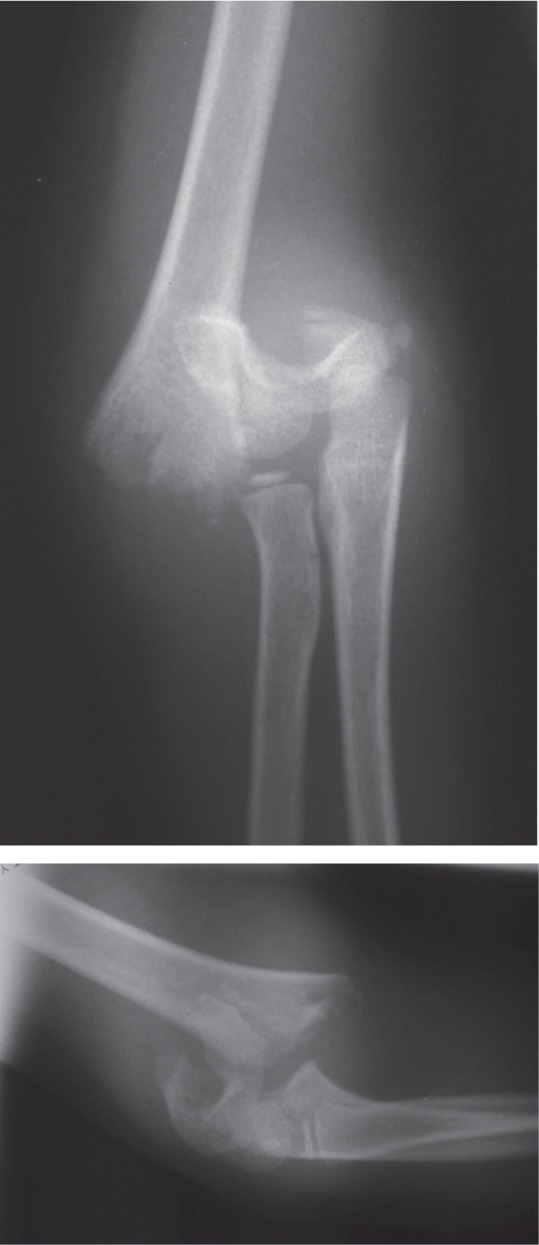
Radiograph showing fracture of the supracondyle of right humerus.
